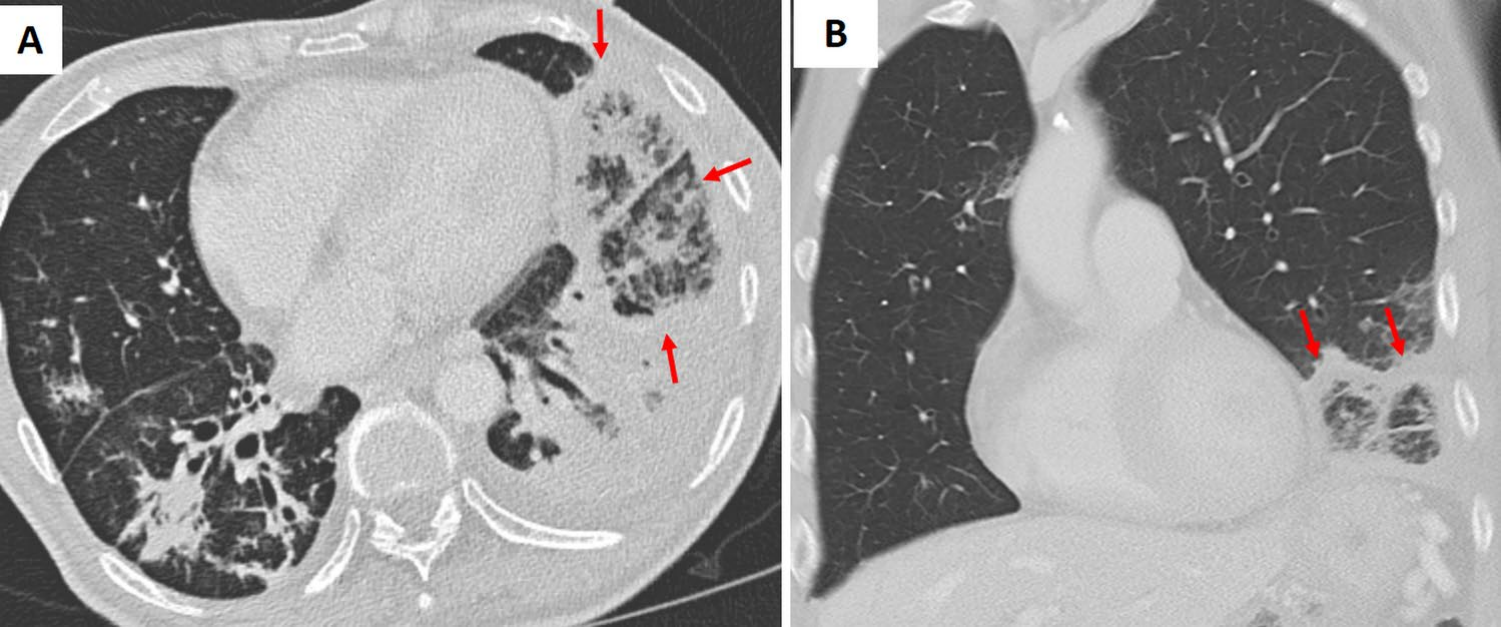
A Axial and (B) coronal chest CT images of a patient with oral squamous cell carcinoma and immunosuppression demonstrate bilateral lower lobe and lingular consolidative pulmonary opacities. The left lower lobe and lingular opacities exhibit the reverse halo (atoll) sign (arrows). Sputum culture confirmed *Staphylococcus aureus* infection
